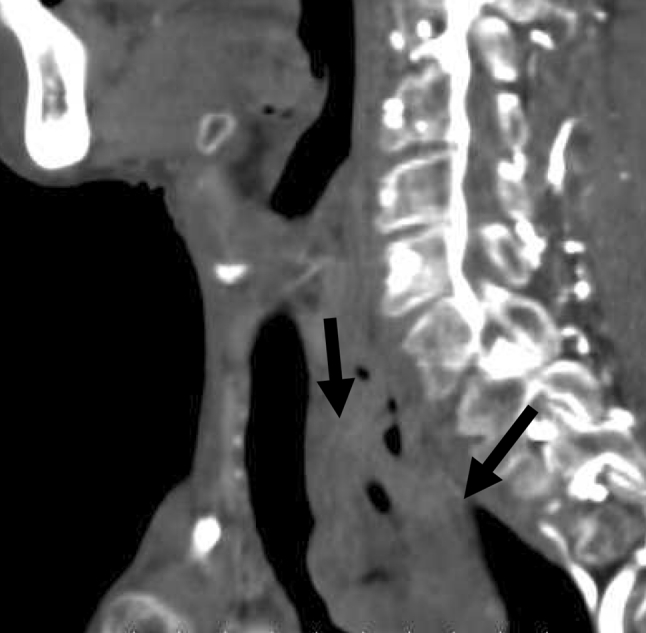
Sagittal CT image obtained with an IVR-CT system immediately before the stent placement demonstrates that cervical esophageal cancer (*arrows*) does not directly invade the hypopharynx
